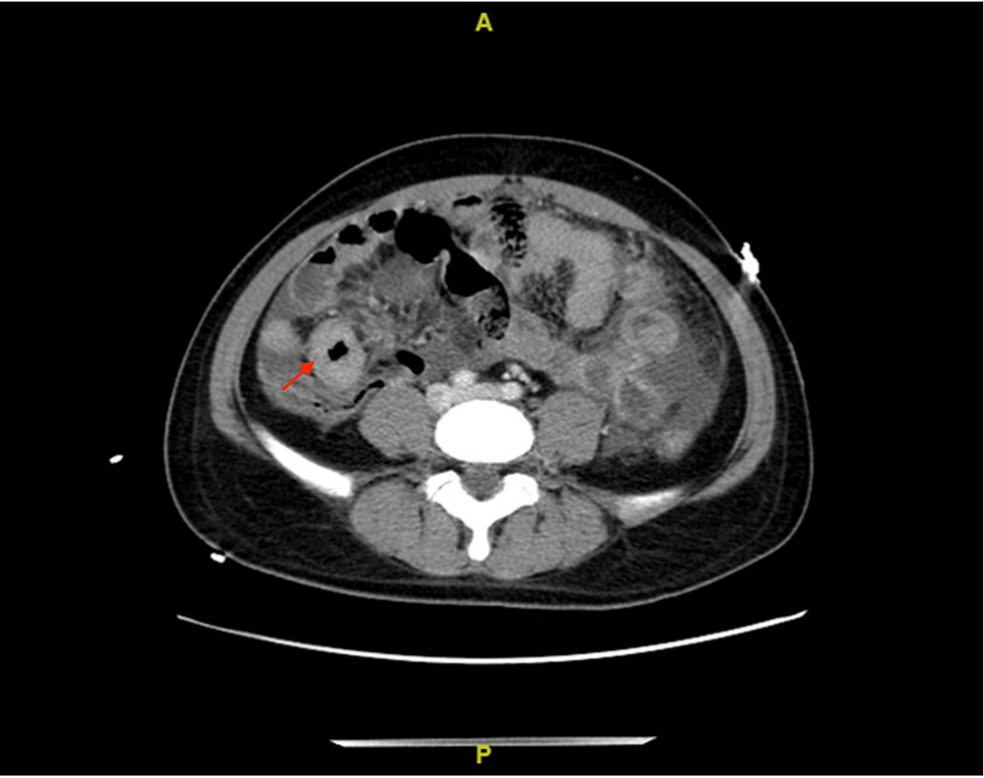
Contrast-enhanced abdomen and pelvis CT revealing full segmental thickening of terminal ileum (red arrow)
